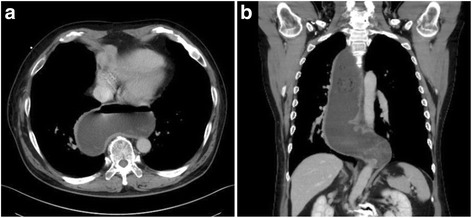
Enhanced CT findings. The esophagus is dilated and the esophagus is meandering, particularly in its inferior portion. a Axial view. b Coronal view
